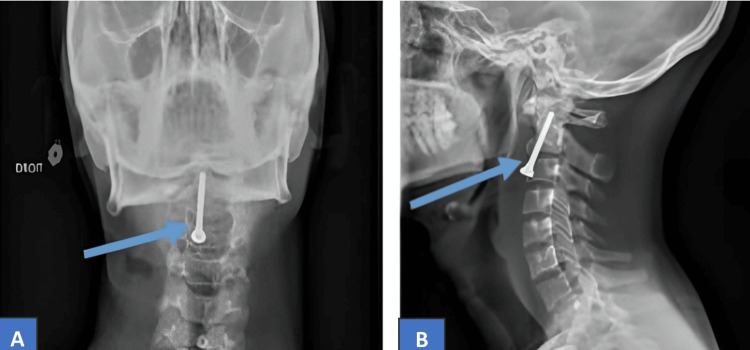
Postoperative X-rays in frontal (A) and profile (B) views show the odontoid screw locations.
